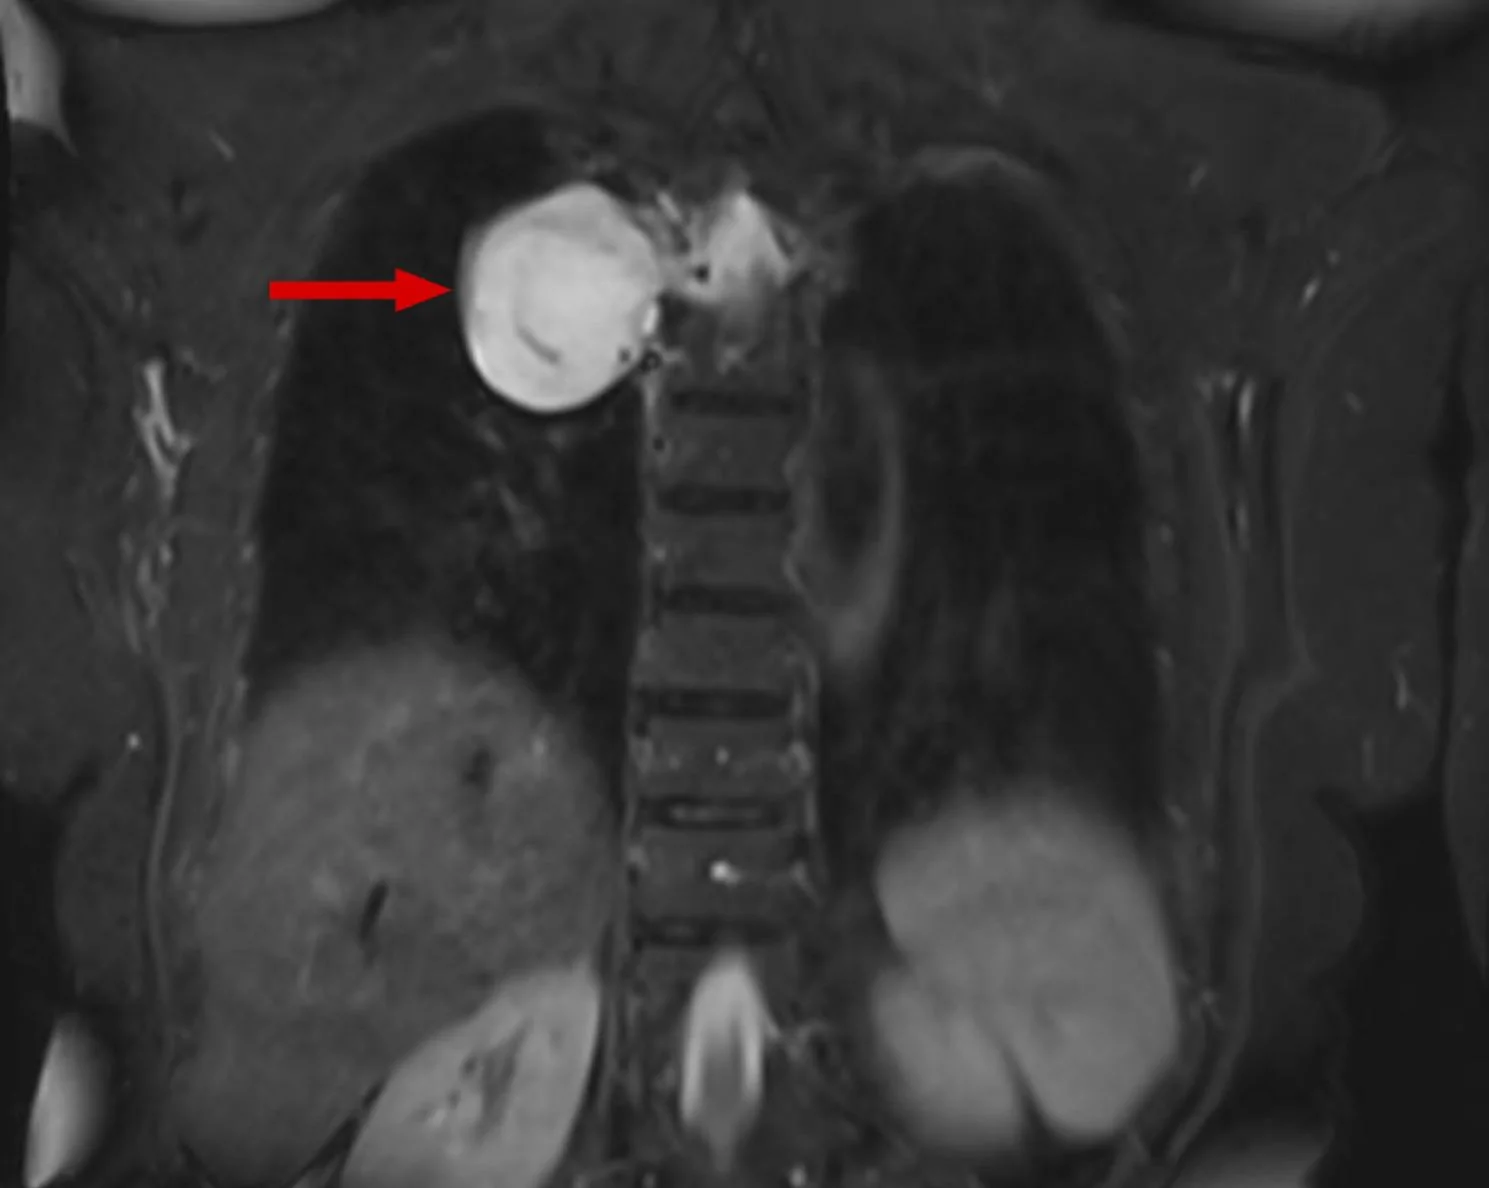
Post-contrast MRI demonstrating the intensely enhancing, dumbbell-shaped lesion (Arrow), highlighting the large extraspinal paravertebral component and the smaller intraspinal component
